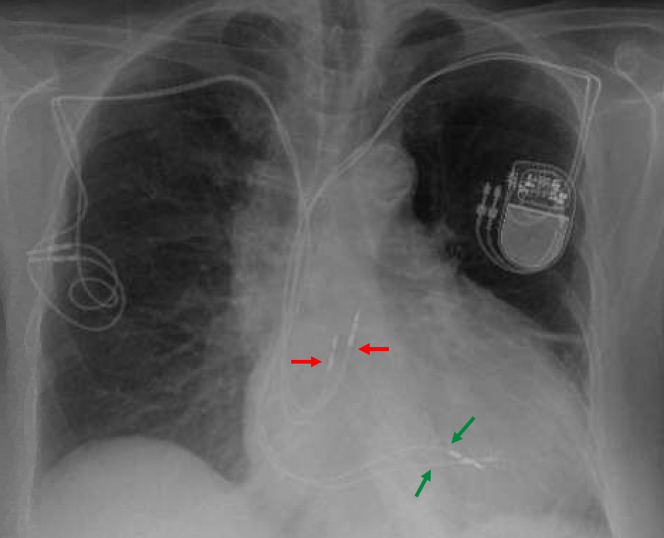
Chest X-Ray After Contralateral Generator Replacement and Implantation of 2 New Leads Anteroposterior chest x-ray after left-sided dual chamber pacemaker replacement and right-sided generator removal showing a total of 2 right atrial (red arrows) and 2 right ventricular (green arrows) leads.
